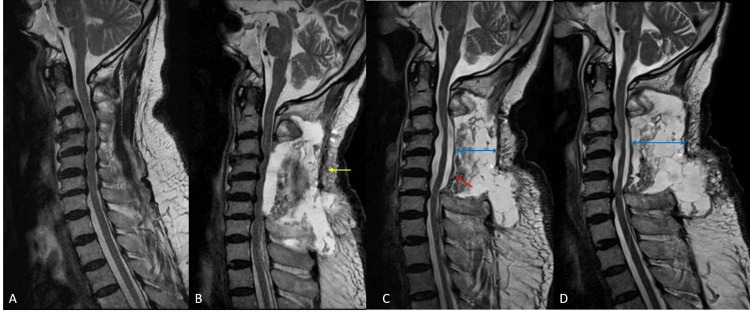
(A) Preoperative MRI demonstrating multilevel cord compression and mild kyphosis. (B) MRI on postoperative day seven demonstrating compressive seroma (yellow arrow). (C) Immediate post-aspiration MRI demonstrating re-expansion of thecal sac (red arrow) following seroma drainage. (D) MRI on post-aspiration day 10 showing a slight increase in the size of the seroma (blue arrows) without significant mass effect on the spinal cord.
